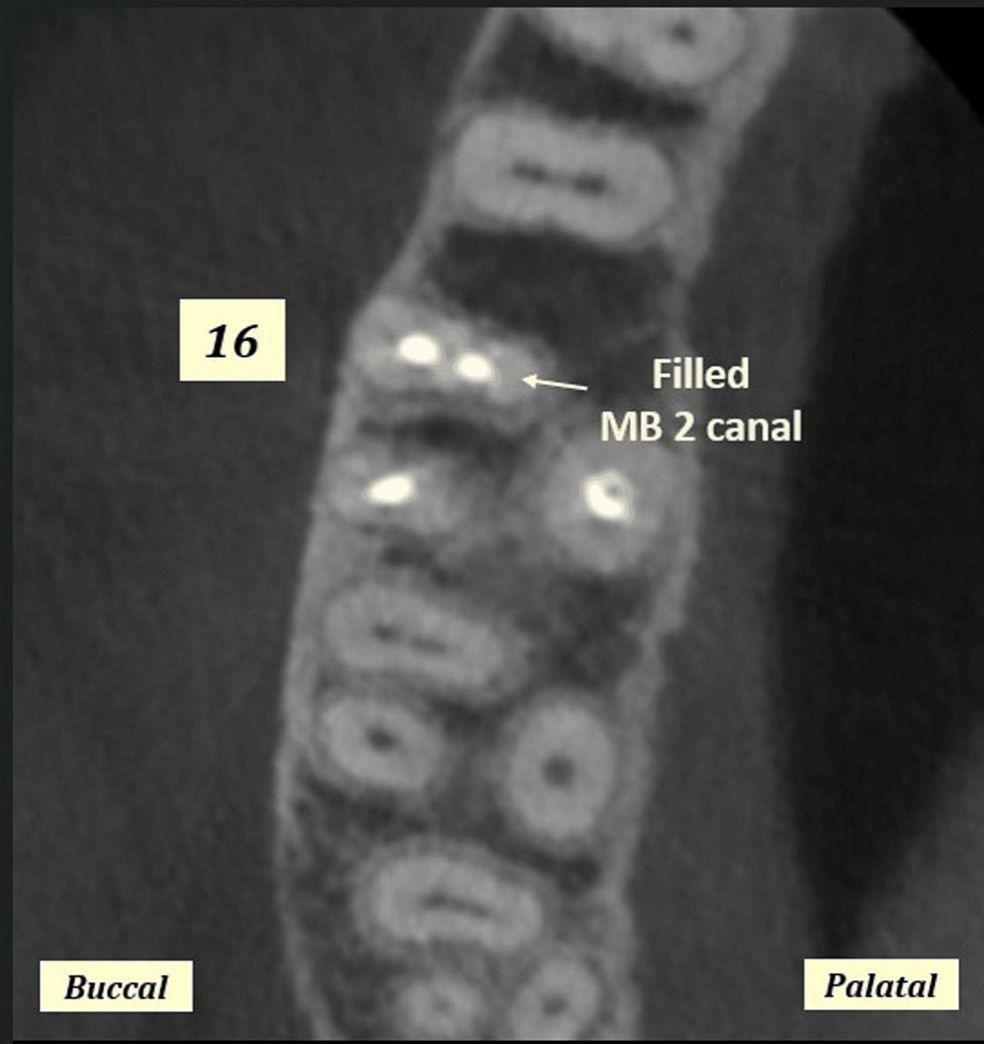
CBCT images showing filled mesiobuccal root canal configurations in axial sections of right maxillary first molars at middle root third level. MB: mesiobuccal; MB2: second mesiobuccal canal; CBCT: cone beam computed tomography
